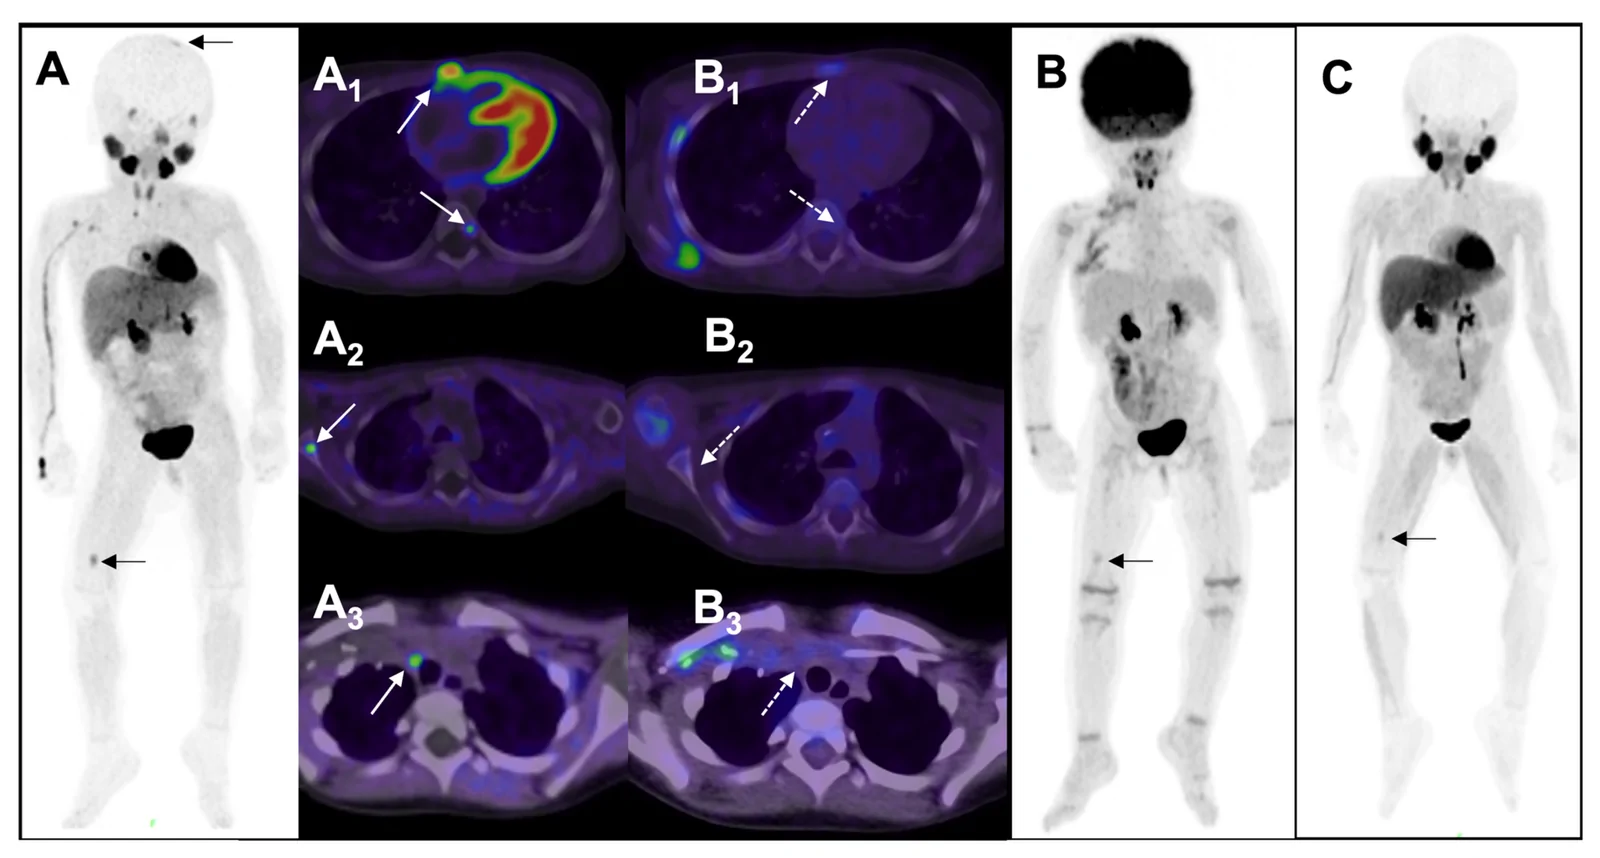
[^18^F]MFBG PET showing occult bone marrow metastases not detected through comprehensive anatomic imaging or [^18^F]FDG PET in newly diagnosed neuroblastoma. A 2-year-old girl with pathologically confirmed differentiating neuroblastoma resected from the mediastinum. Comprehensive anatomic imaging revealed no evidence of metastatic disease. Bone marrow biopsy and genetic testing (including MYCN and 11q status) were negative. [^18^F]MFBG PET/CT MIP (A) demonstrates multiple foci of abnormal uptake (arrows). Axial PET/CT fusion images show additional MFBG-avid lesions in the sternum and T7 vertebra (A₁), right scapula (A₂), and anterior chest wall adjacent to the prior surgical site (A₃), none of which demonstrate corresponding structural abnormalities on CT. Corresponding [^18^F]FDG PET/CT MIP (B) and fusion images (B₁–B₃) show no abnormal uptake at these sites. Based on [^18^F]MFBG PET findings, INRG stage was revised from L2 to M, and risk classification escalated from intermediate to high risk. (C) Follow-up [^18^F]MFBG PET MIP after high-risk induction therapy demonstrates partial response.
